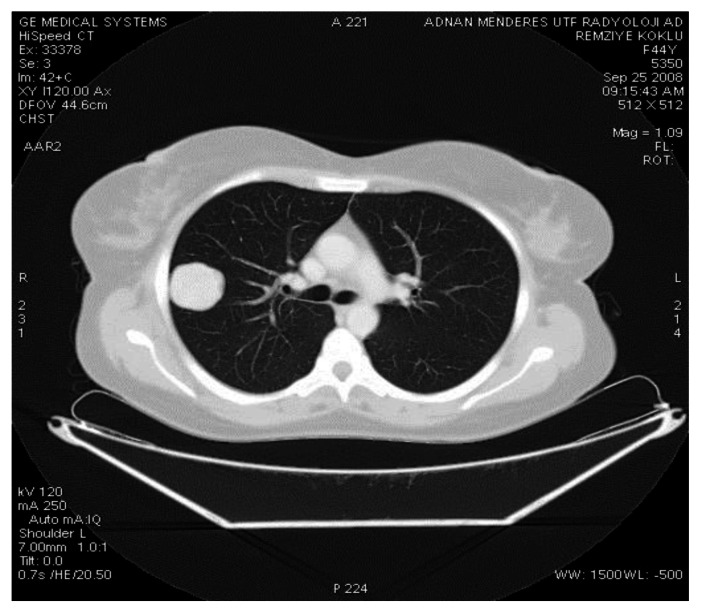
In CT parenchyma window in right lung upper lobe apical segment, a solid peripheral subpleural situated nodule which is 37 mm in diameter and has a homogeneous formation showing umbilication in lobule with a contour-good bordered medial part.
